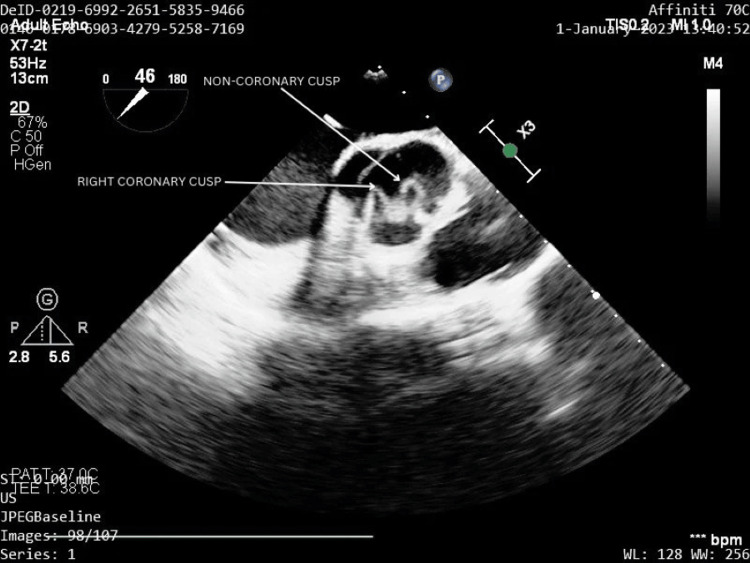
Prolapse of the non-coronary cusp and the right coronary cusp of the aortic valve seen on transesophageal echocardiography (marked with a white arrow). The above image has been taken by the authors.
